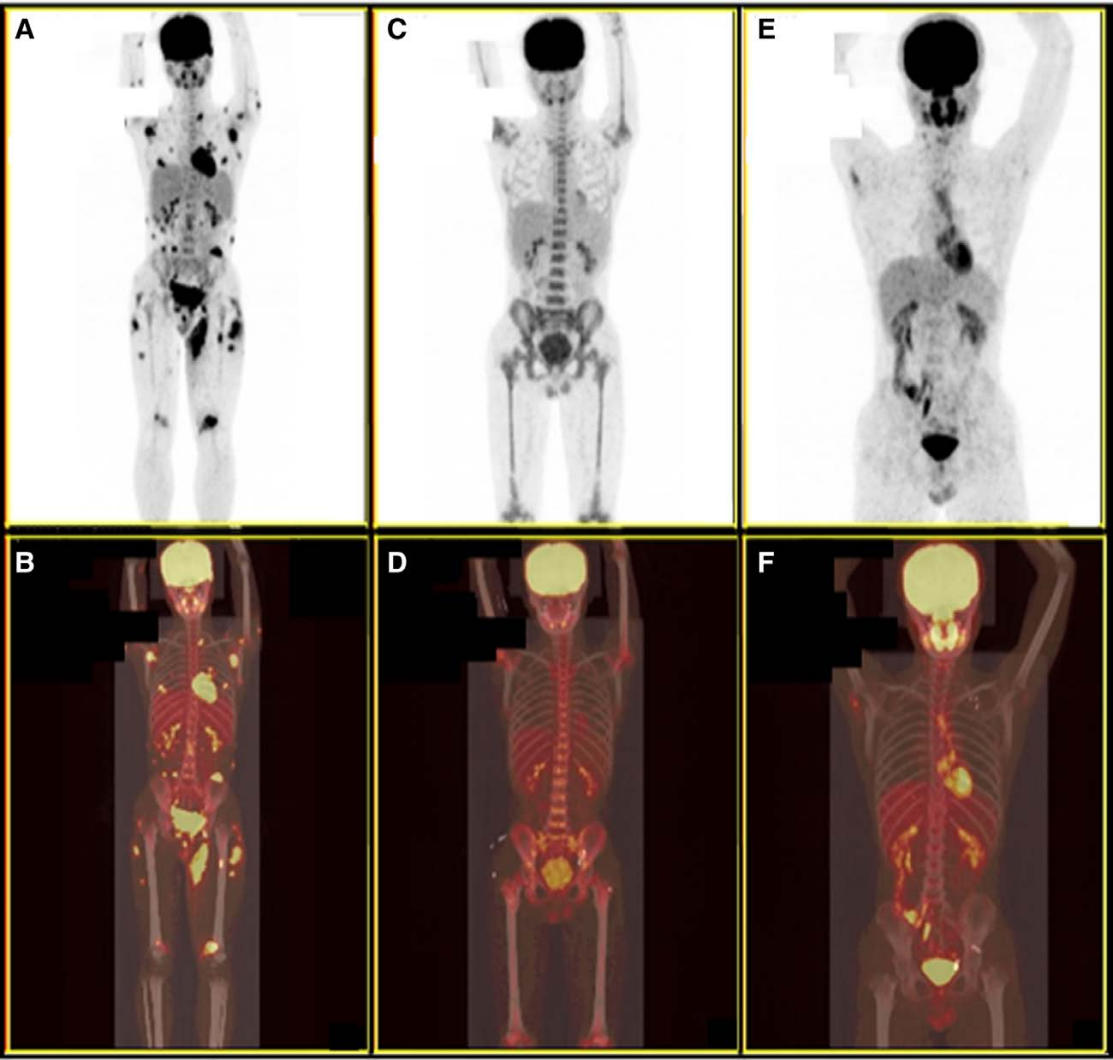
PET/CT images of the SPTCL patient at different time points. (A–F) PET/CT images of this case at the initial diagnosis (A and B), after 8 cycles of chemotherapy (C and D) and 16 months after auto-HSCT (E and F). auto-HSCT = autologous hematopoietic stem cell transplantation, PET/CT = positron emission tomography/computed tomography, SPTCL = subcutaneous panniculitis like T-cell lymphoma.
